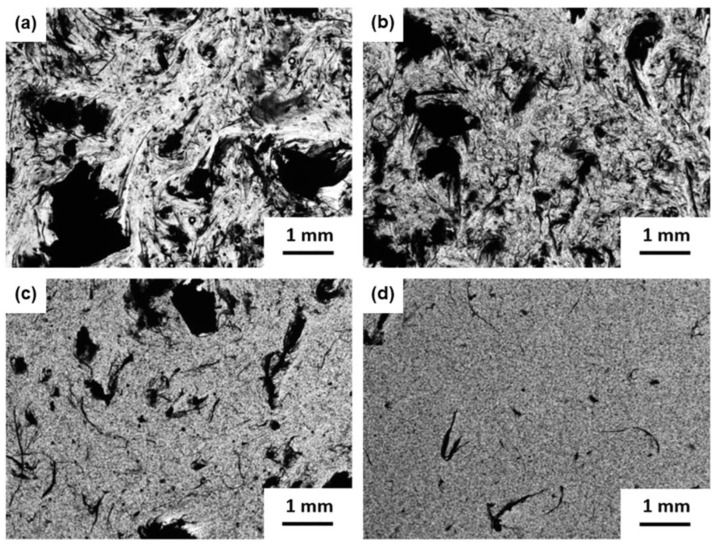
Optical images of (a) the epoxy/SWCNT (I_d_ = 0.104 ± 0.007), (b) epoxy/GNP_2_SWCNT_8_ (I_d_ = 0.108 ± 0.01), (c) epoxy/GNP_5_SWCNT_5_ (I_d_ = 0.201 ± 0.021) and (d) epoxy/GNP_8_SWCNT_2_ (I_d_ = 0.443 ± 0.027) mixtures after sonication in ultrasonic bath at 80 °C for 90 min.
